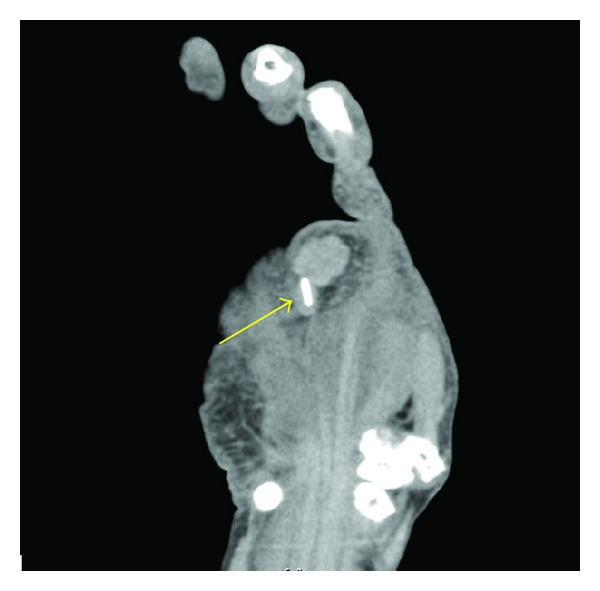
Contrast-enhanced CT showing a linear radiopaque structure of 8 mm long with a mass on its distal end.
